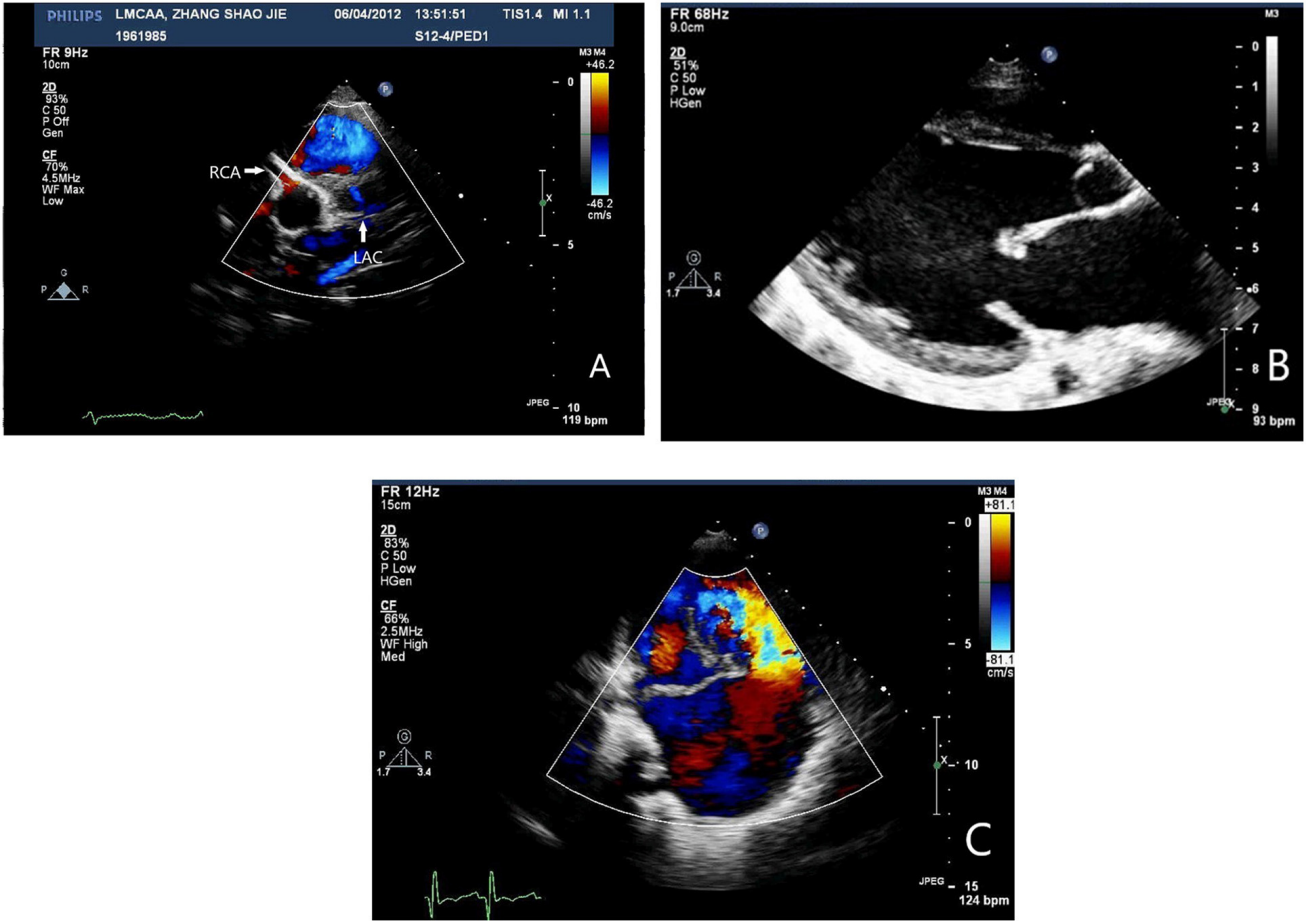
(A) Echocardiography: No left coronary artery opening in the left coronary sinus, the origin and course of RCA is normal. RCA dilated the signal of reverse perfusion blood flow can be seen in the slender left main coronary artery. (B) Echocardiography: Echo enhancement of mitral valve, papillary muscle, and endocardium. (C) Echocardiography: Massive mitral regurgitation.
